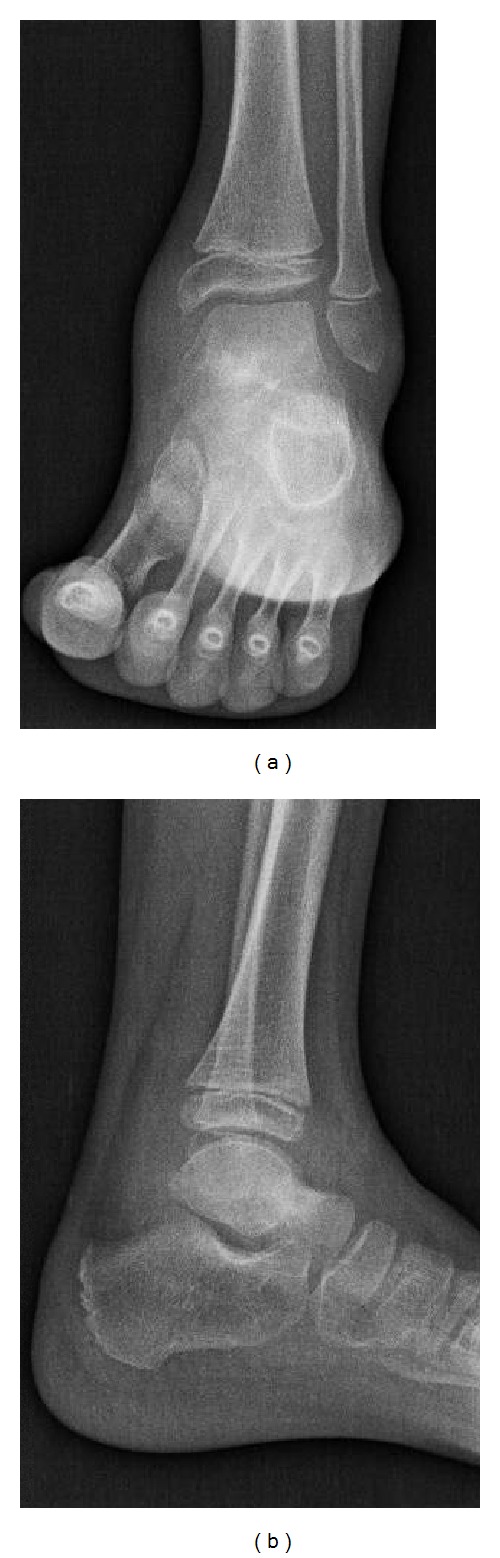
8-year-old boy with osteoid osteoma of the left talar neck. Synovial thickening of the ankle and subtalar joint was diagnosed with echography. X-ray showed ankle effusion but no bone anomaly was initially reported. However retrospective X-ray analysis showed a talar neck hyperostosis.
